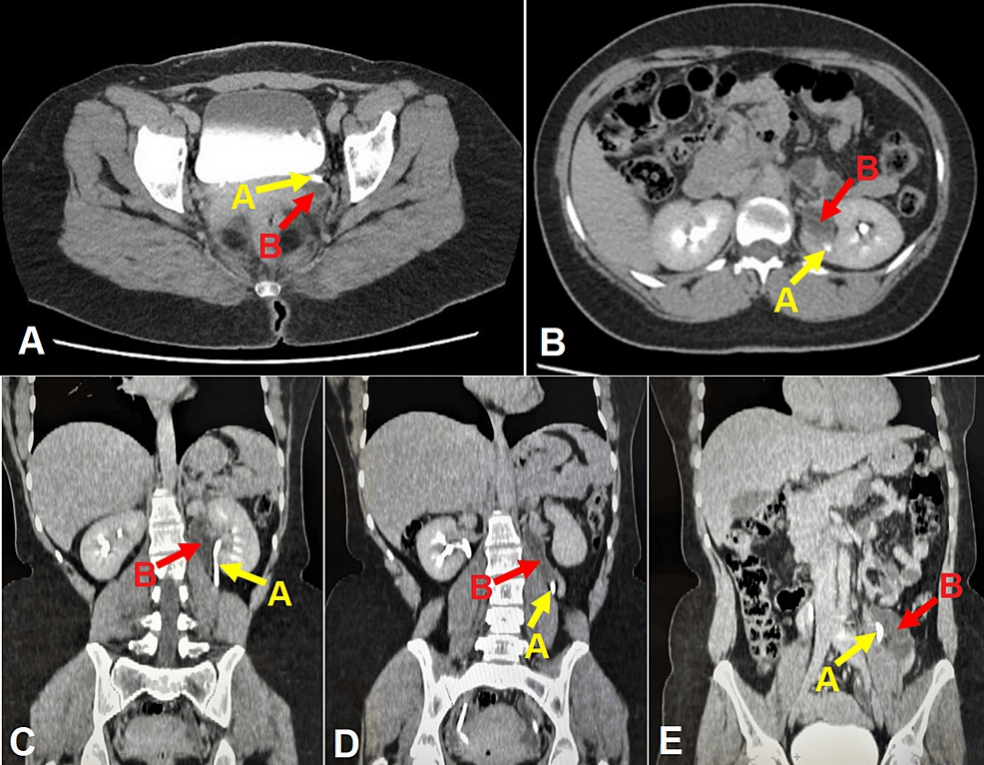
Computed tomography showing duplicated pyelocaliceal system and complete duplication of the ureter on the left side, with dilated ectopic ureter associated with consequent parenchymal atrophy of the upper pole of the left kidney. (A) and (B) A (yellow arrow) indicates a ureter of normal caliber and B (red arrow) an enlarged ectopic ureter; (C)-(E) red arrow B indicates the proximal, medial, and distal ends of the ureteral duplication, respectively, and yellow arrow A indicates the normal ureter.
